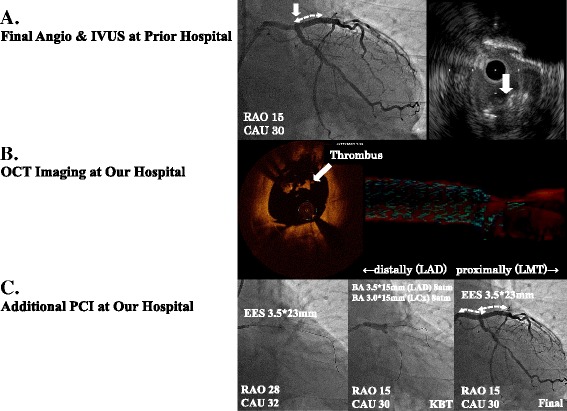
a Final left coronary angiography (CAG, right anterior oblique caudal view) at prior hospital did not show any significant obstruction from proximal left anterior descending coronary artery (LAD) to left main trunk (left panel). A stented segment is shown in white double-headed dashed arrow. Intravascular ultrasound (IVUS) image at just proximal LAD clearly demonstrated deformed stent struts protruding toward the lumen (white arrowhead), however, adhesion of thrombus to the struts was ambiguous on the IVUS image (right panel). The site of stent deformation was indicated by white arrow head on CAG (left panel). b Optical coherence tomography (OCT) imaging around the deformed struts performed at our hospital clearly showed thrombus adhesion to the deformed struts with radial signal attenuation behind the adherent structure to the struts (white arrowhead, left panel). 3D reconstruction image from proximal LAD to left main trunk (LMT) visualized fractured struts extending to the LMT direction shown in light blue (right panel). c Additional percutaneous coronary intervention underwent at our hospital. A 3.5x23mm EES was implanted from LMT to LAD (left panel). Kissing balloon technique using 3.5x15mm and 3.0x15mm non-compliant balloons were performed (middle panel). Final CAG after additional stenting followed by KBT (right panel). A stented segment is shown in white double-headed dashed arrow
